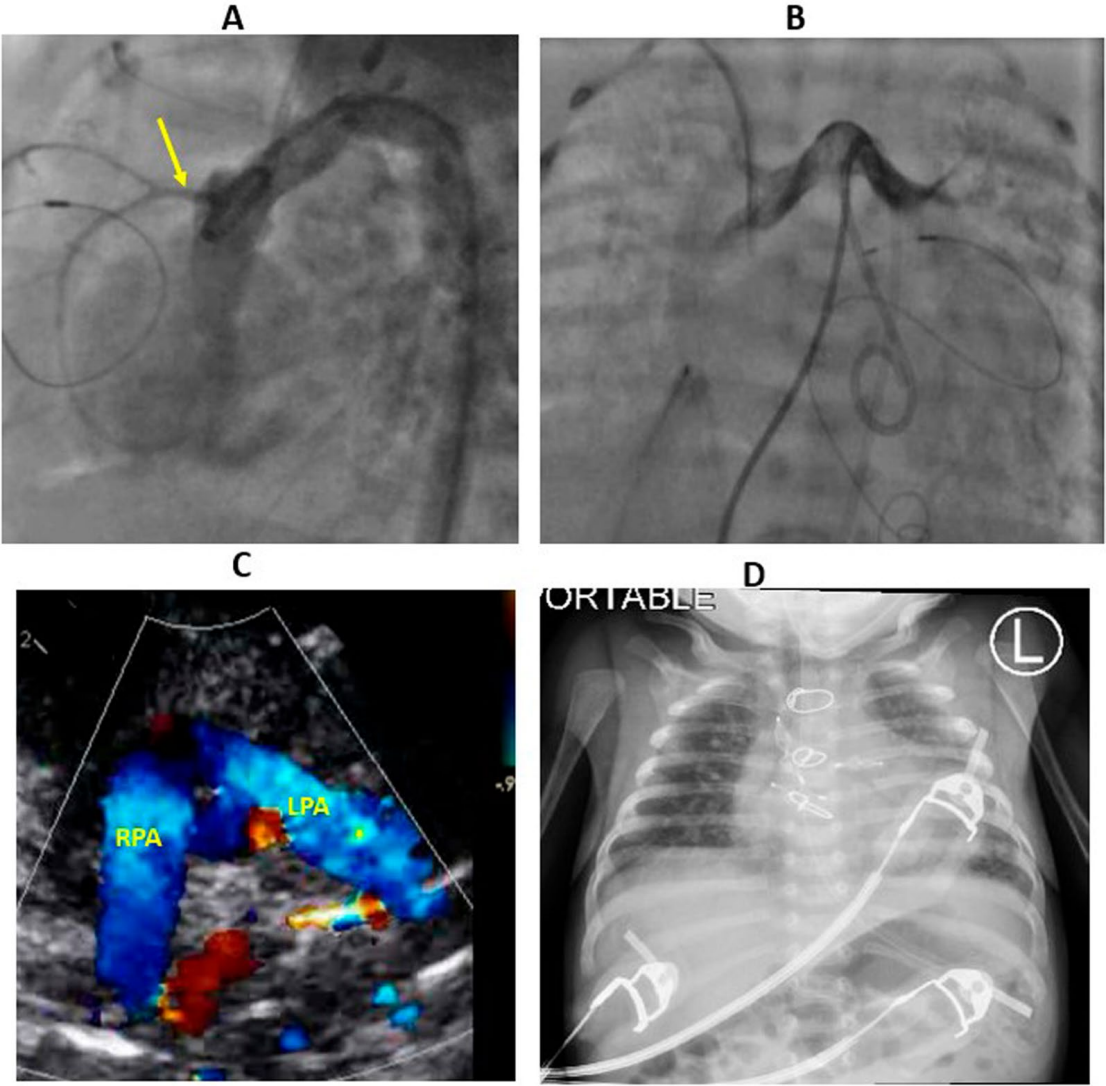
Roentgenogram, angiography, and echocardiography after ASO. A aortic angiography showing normal right coronary artery (yellow arrow). B Pulmonary angiography showing patent pulmonary arteries. C Parasternal short axis echocardiographic view showing the Lecompte with patent pulmonary arteries. D chest X-ray is done after successful closure of MAPCAs, showing improvement of pulmonary edema and skin edema. ASO: arterial switch operation, MAPCAs: Major aortopulmonary collateral arteries. *RPA* right pulmonary artery, *LPA* left pulmonary artery
